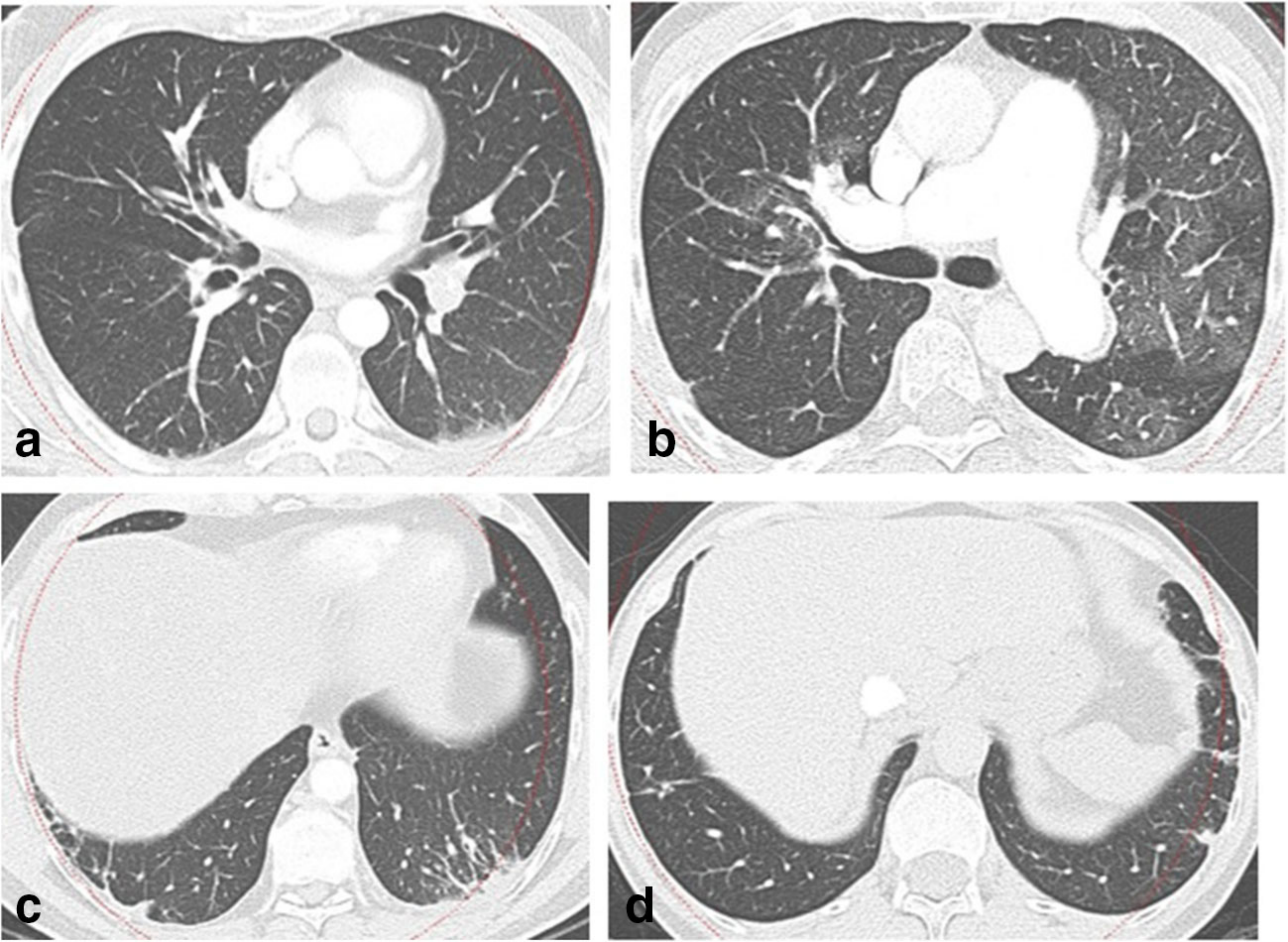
Lung window (b80 kernel reconstruction) CT. Lung hyperaemia is well evident in CTEPH (b) with both central and peripheral distribution, whereas is absent in CTEPD (a). Peripheral scars are present in both CTEPD (c) and CTEPH (d) patients
